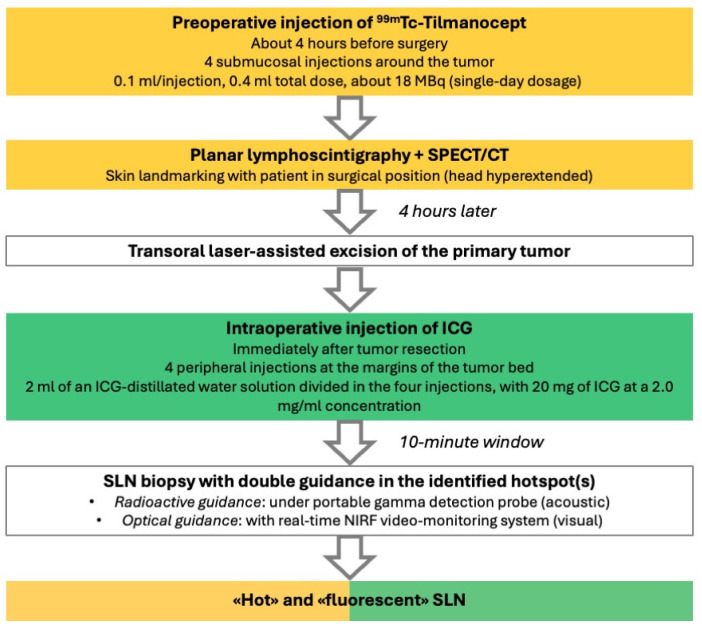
A stepwise description of the proposed multimodal protocol for sentinel lymph node biopsy with double guidance. SPECT/CT: single-photon emission computed tomography/computed tomography; ICG: indocyanine green; SLN: sentinel lymph node.
